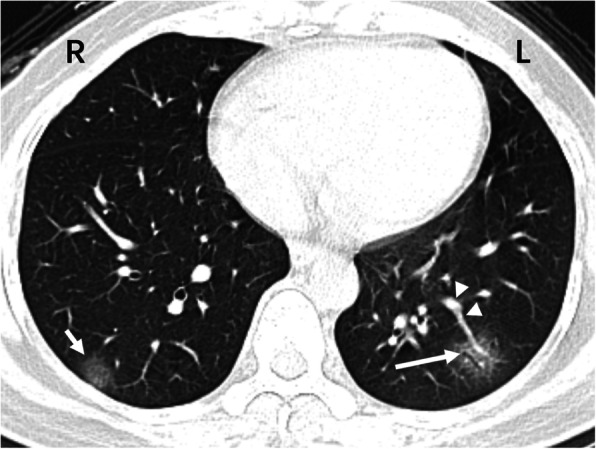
This CT image refers to the same patient (F, 39Y) as Figure 3. Patchy GGO is seen in the posterior basal segment of the left lower lobe and the posterior basal segment of the right lower lobe. The right lung lesions are localized in the peripheral lung field adjacent to the subpleural region (short white arrow). The outermost edge of the left lung lesions is 3.7 mm from the subpleural areas, and there are visible air-filled bronchi (long white arrow) inside the lesion; the white arrowheads show that a vein in the lower left lung has penetrated the lesion. R = Right, L = Left
